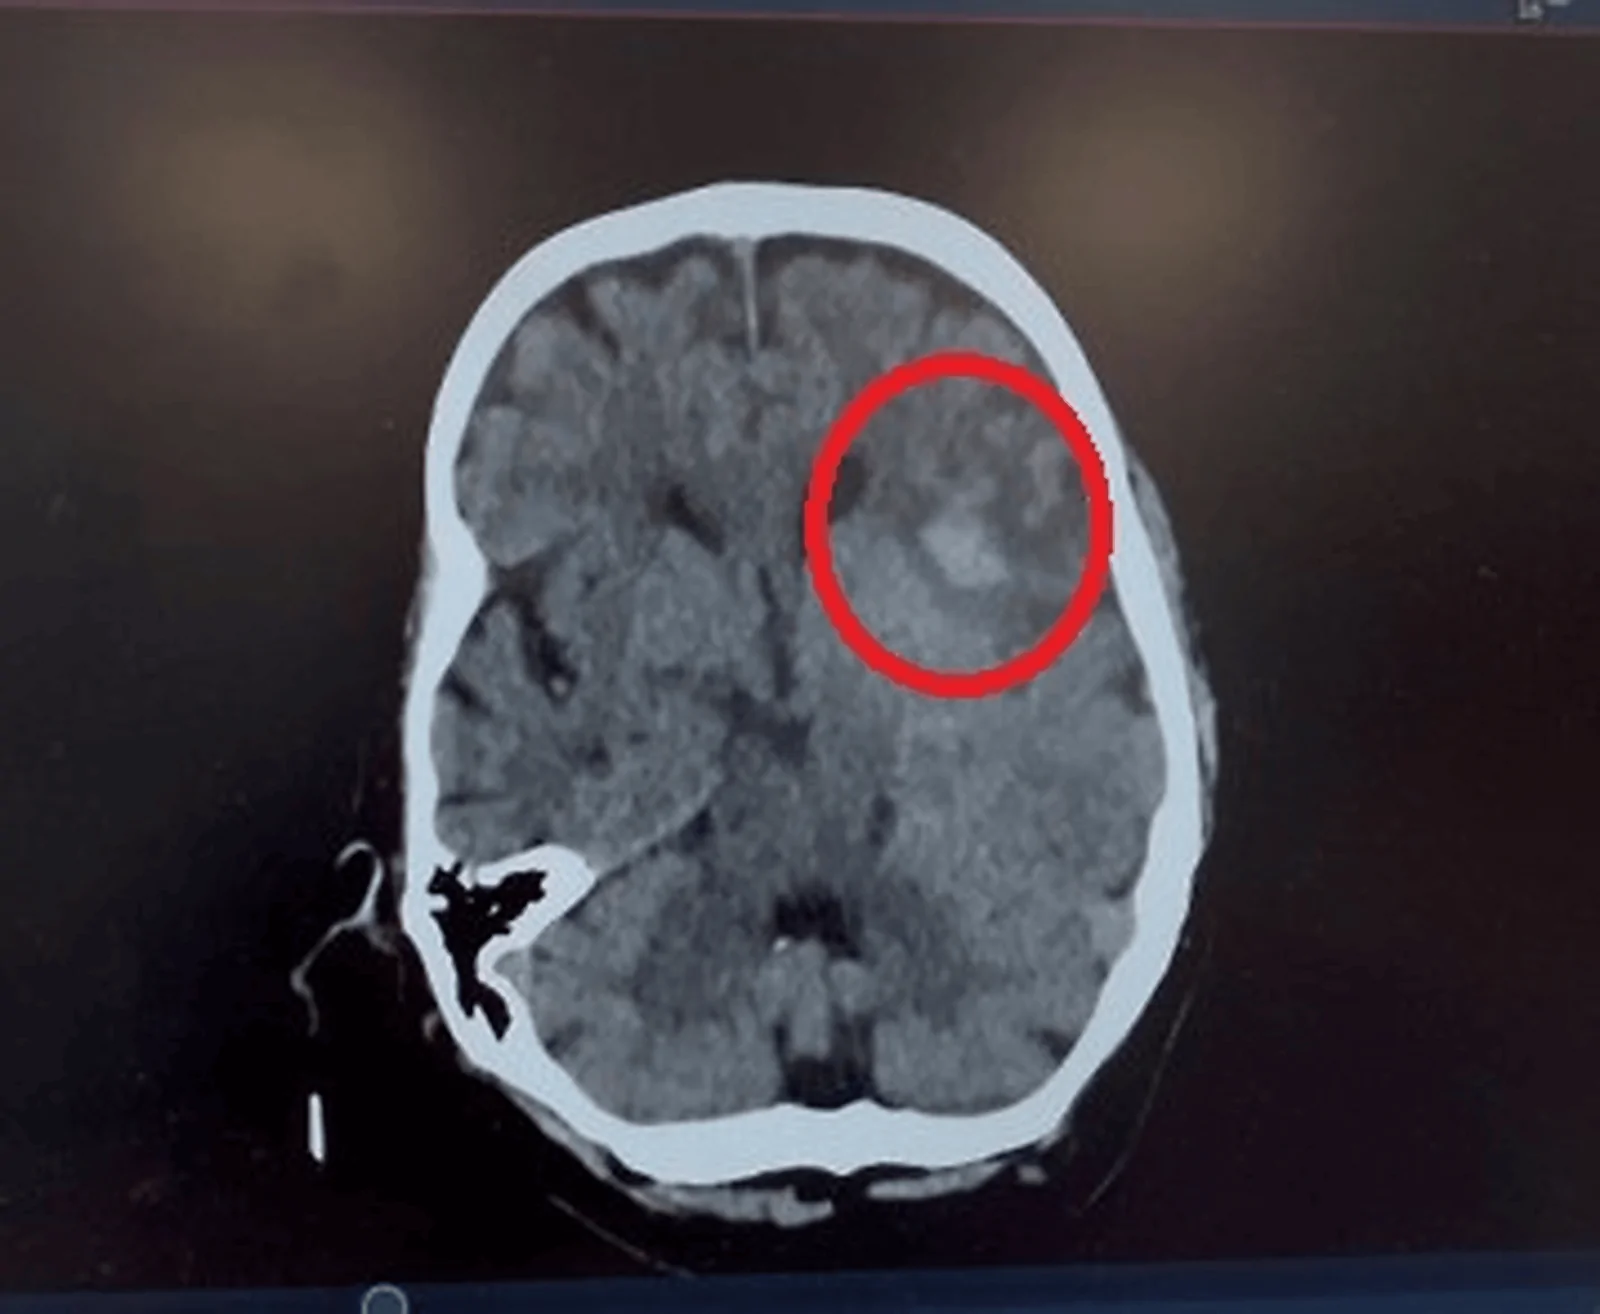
Post-thrombolysis and thrombectomy non-contrasted CT brain Post-reperfusion non-contrast CT brain demonstrating focal hemorrhagic transformation within the left middle cerebral artery infarct territory (red circle), without significant mass effect or midline shift.
